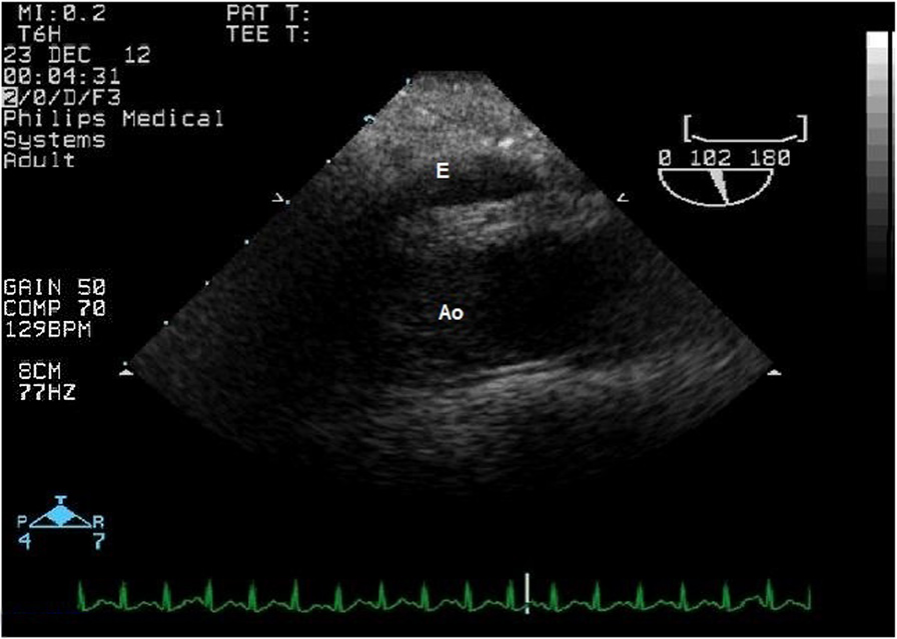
Transesophageal echocardiography. Descending aorta – long axis view. Increased distance between the probe and the aortic wall with the echo-free space suggestive for the para-aortic effusion. Ao – aorta, E - effusion.
